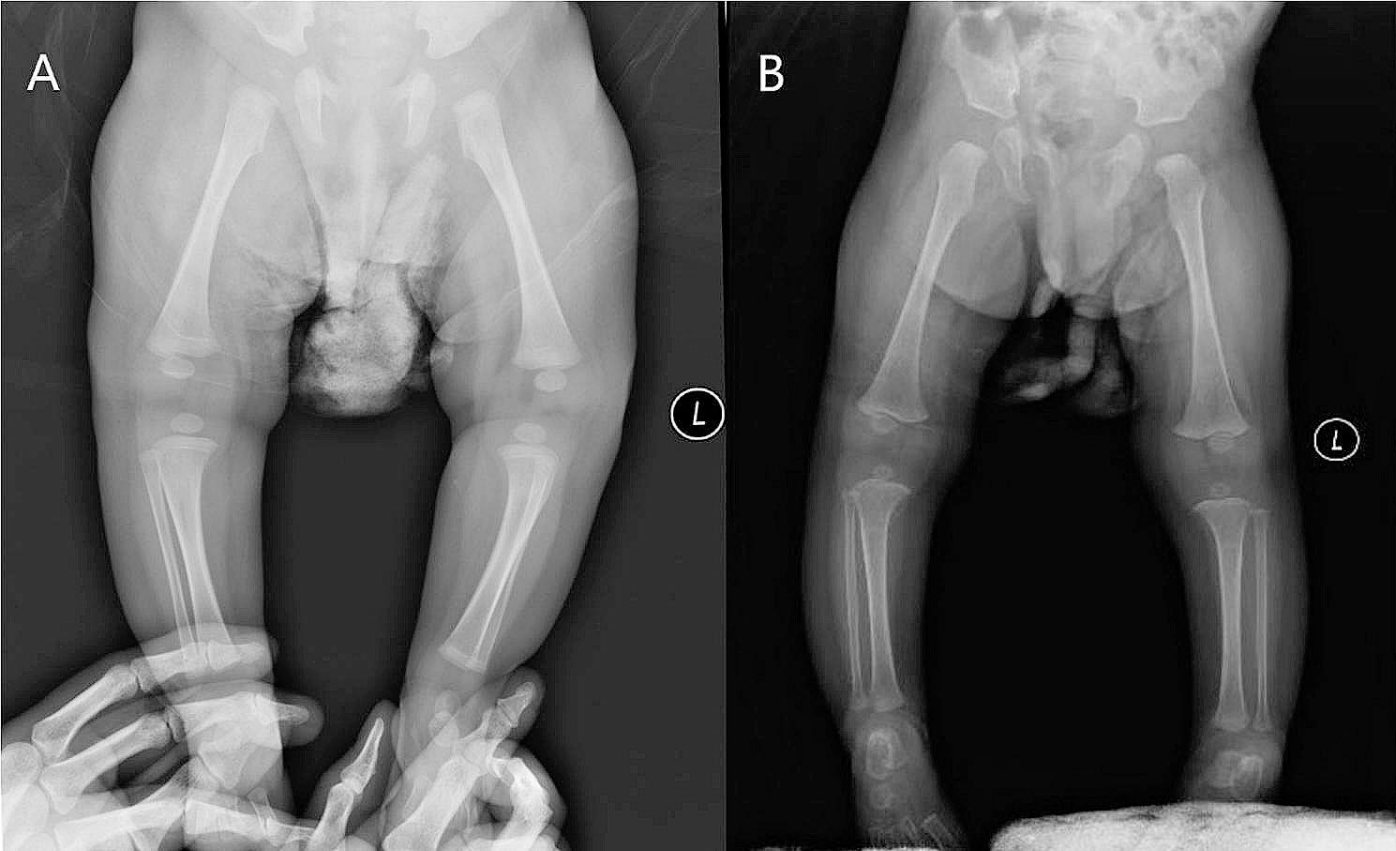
X-ray examination of limbs. (A) Long bone panorama of Patient 3 showed dense transverse banded shadows in Bilateral distal femur and tibial metaphysis. (B) Radiography of Patient 5 showed temporary calcification zone is slightly narrow and dense of in the metaphysis of the bone
